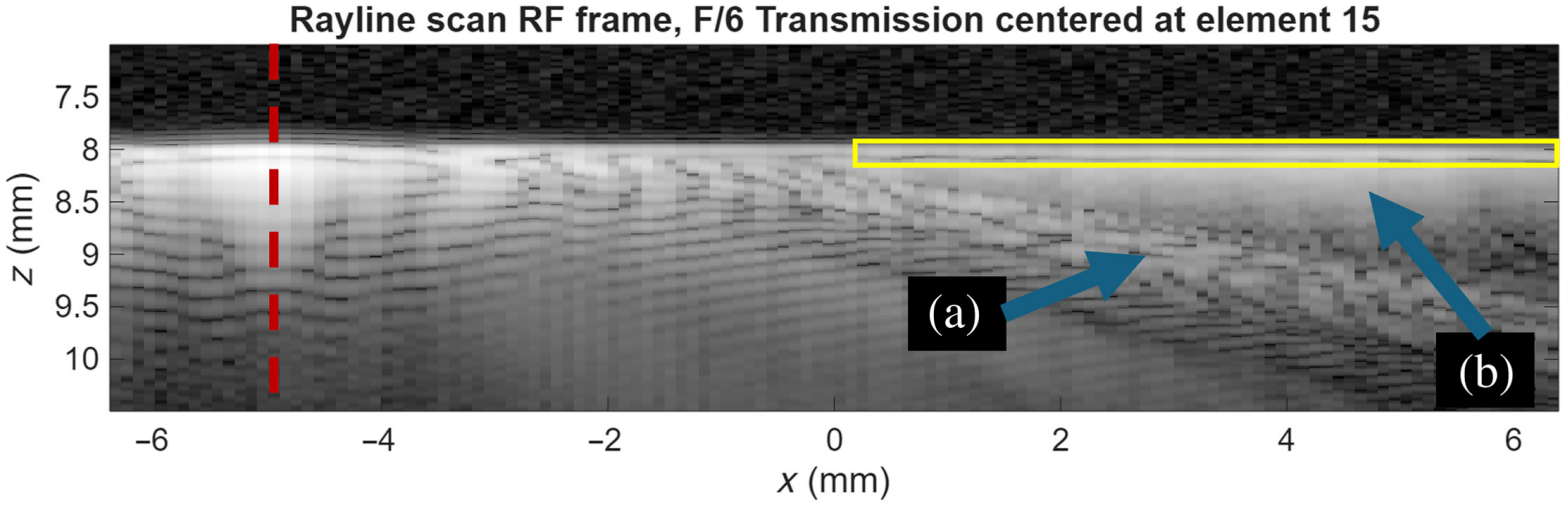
RF frame from a rayline scan aperture with transmission aperture index 15, with the red dashed line representing the rayline axis and the yellow box representing an example ROI to analyze intensity from optical window ringing. (a) Expected reflection from the incident ultrasound pulse. (b) Unexpected off-axis intensity due to the optical window ringing.
